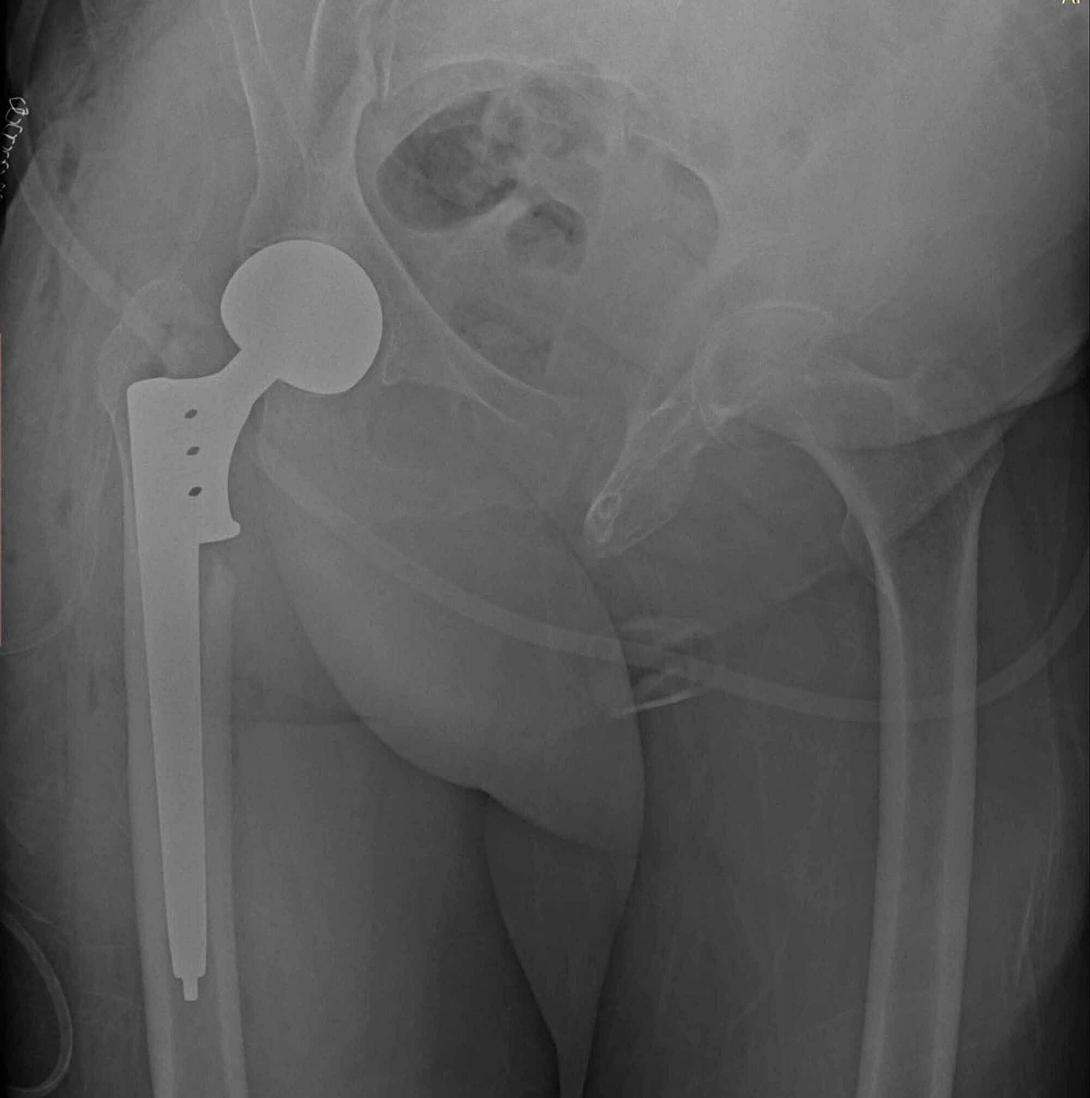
Postoperative first-day anterior-posterior hip radiography
